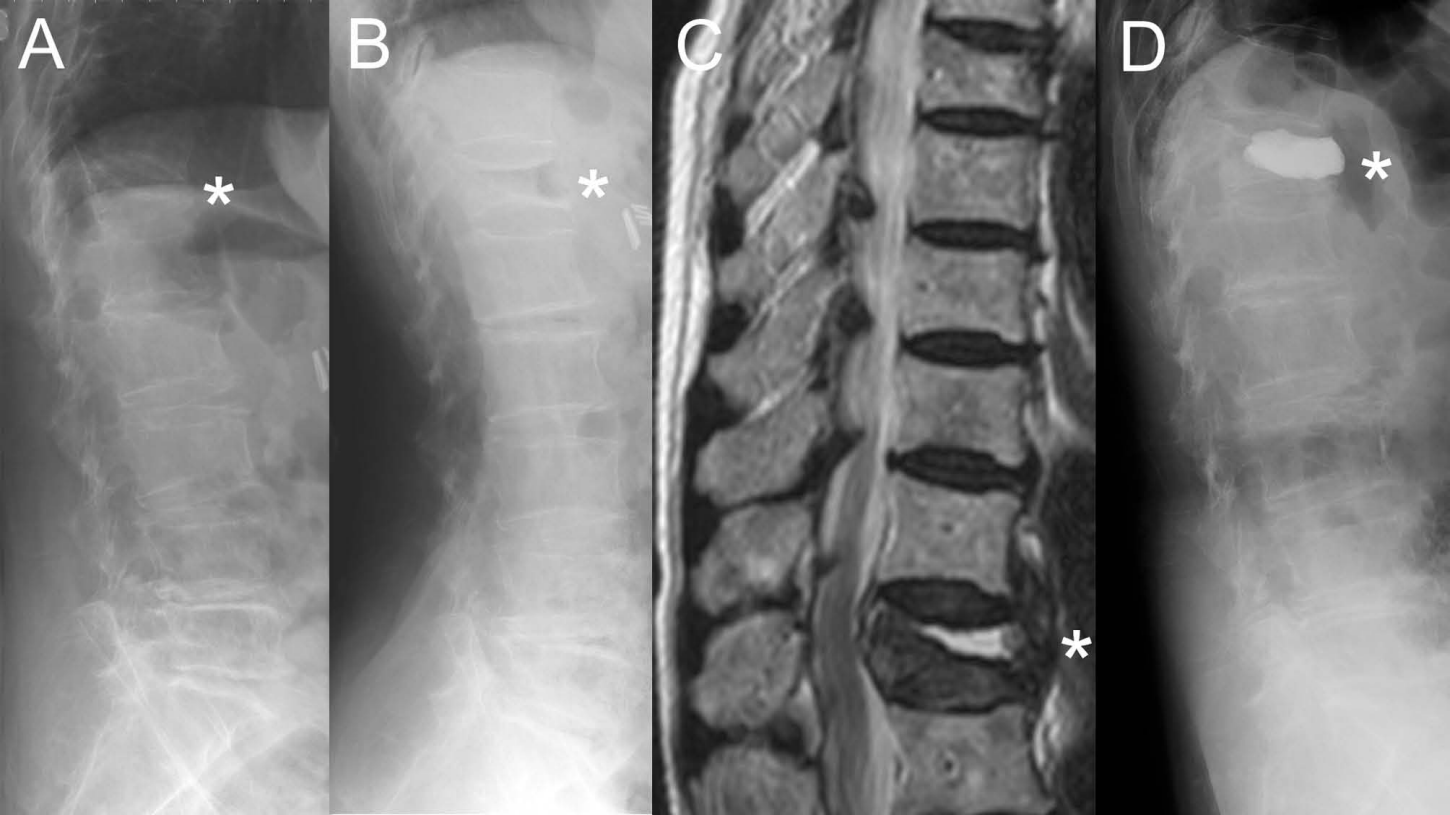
The intravertebral cleft detected on the supine lateral radiograph. A 93-year-old male patient with T12 severely collapsed vertebral compression fracture (VCF) with preoperative VAS score of 9, vertebral height (VH) ratio of 24.5%, and a kyphotic angle of 31.9° on the preoperative standing lateral radiograph (StLR) (A). The supine lateral radiograph (SuLR) (B) revealed an intravertebral cleft (IVC) and the VH ratio and kyphotic angle were reduced to 39.9% and 14.1°, respectively. We performed T2-weighted MRI (C) to confirm the level of symptomatic VCF at 12 weeks after a fall from standing height, which showed an IVC with fluid sign. The postoperative StLR (D) showed that the VH ratio and kyphotic angle were restored to 60% and 24.3°, respectively. The VAS score of this patient decreased to 3, 1, and 0 points at day 1, 1 month, and 4 months, respectively, post operation. (* T12 vertebra)
